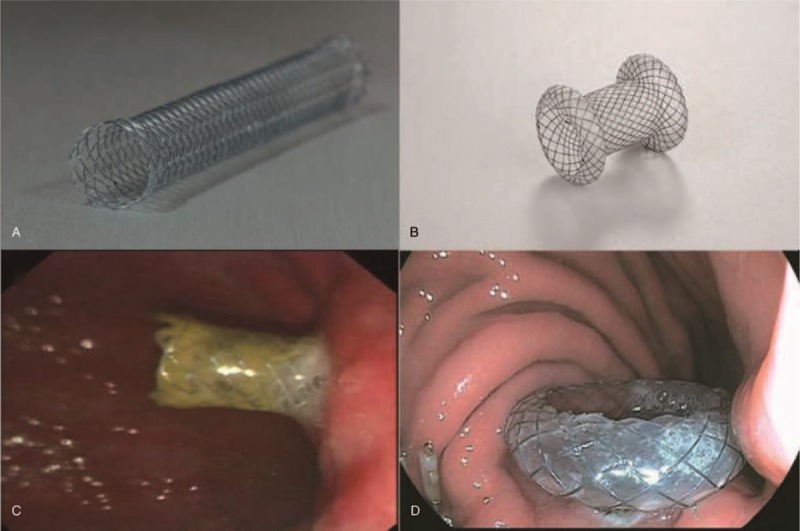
Endoscopic ultrasound-guided transmural placement of self-expanding tubular metal stent (A, C) and biflanged metal stent (B, D) for drainage of pancreatic pseudocyst.
